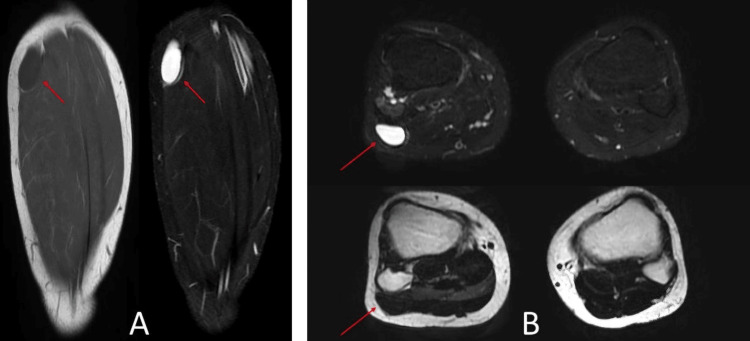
MRI findings Multi-planar multi-sequential MRI with contrast. (A) Coronal MRI of the right leg showing the lesion (red arrow). (B) Axial MRI of both legs with the lesion indicated by the red arrow MRI: magnetic resonance imaging
